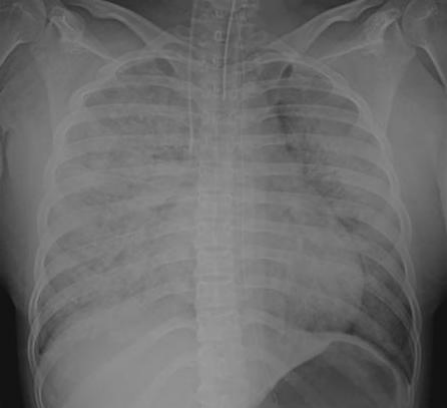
The patient's chest X-ray on admission to the intensive care unit. Diffuse infiltration was observed throughout the lungs.
